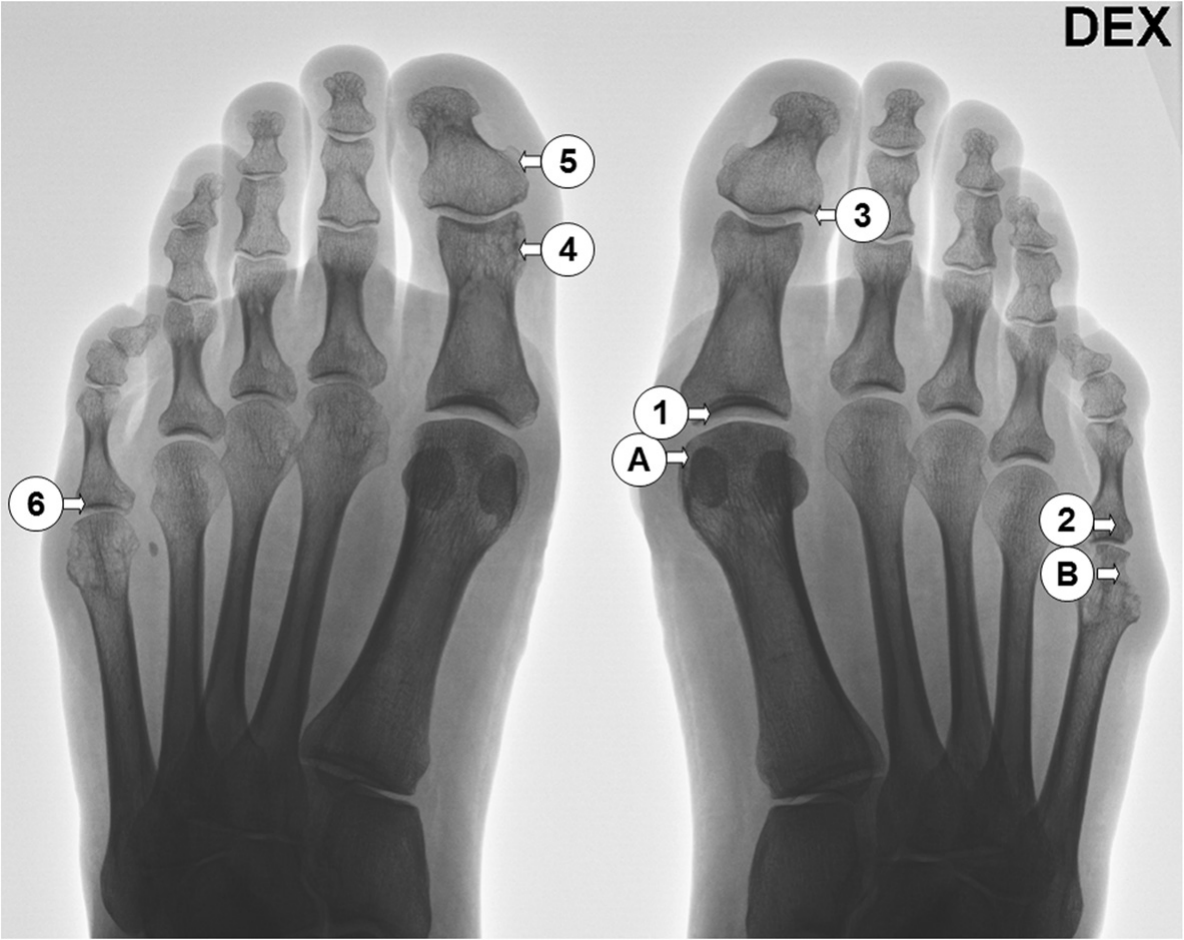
The patient radiograph taken before the first surgery. The locations of the samples taken are presented by A and B for the first operation and numbered from 1–6 for the second operation.
